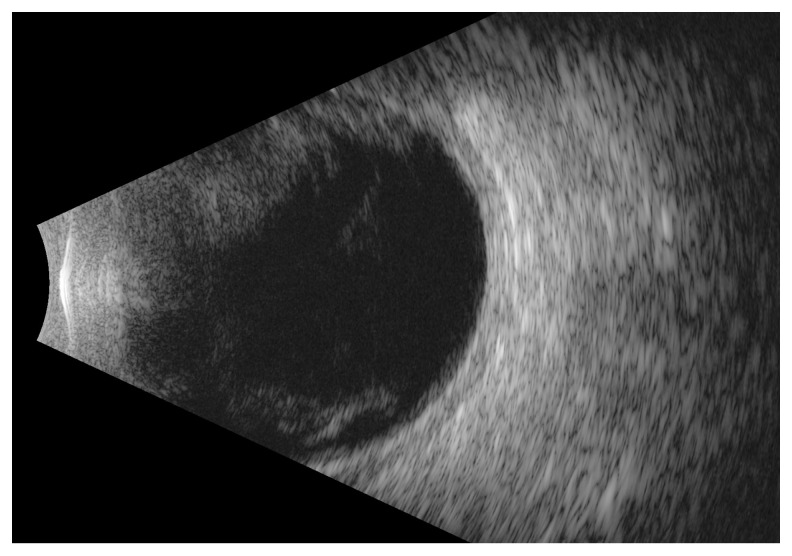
B-scan ultrasound of the left eye shows hyperechoic material in the vitreous chamber on admission.
